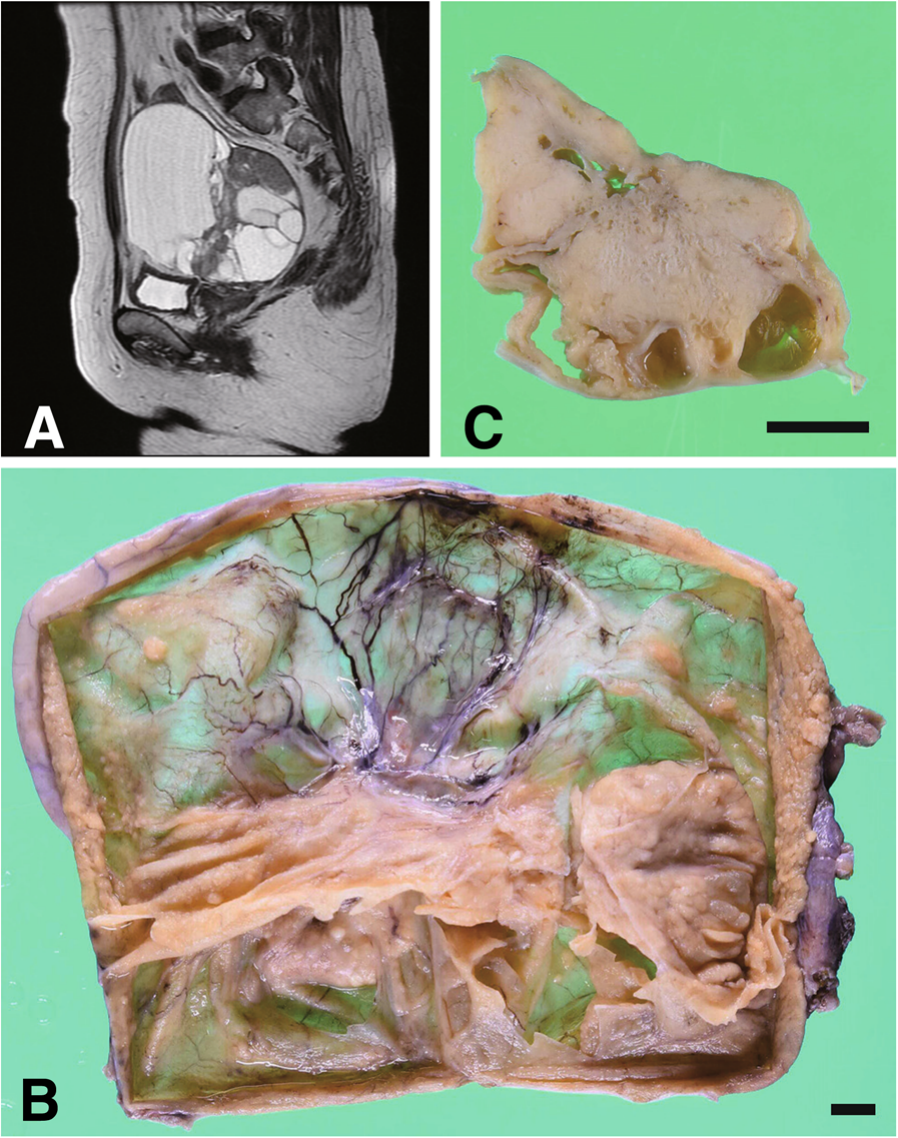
Overview of the ovarian cancer. a Representative sagittal view of magnetic resonance imaging. The inside (b) and cut surface (c) of the ovarian tumor. Black bars: 1 cm
